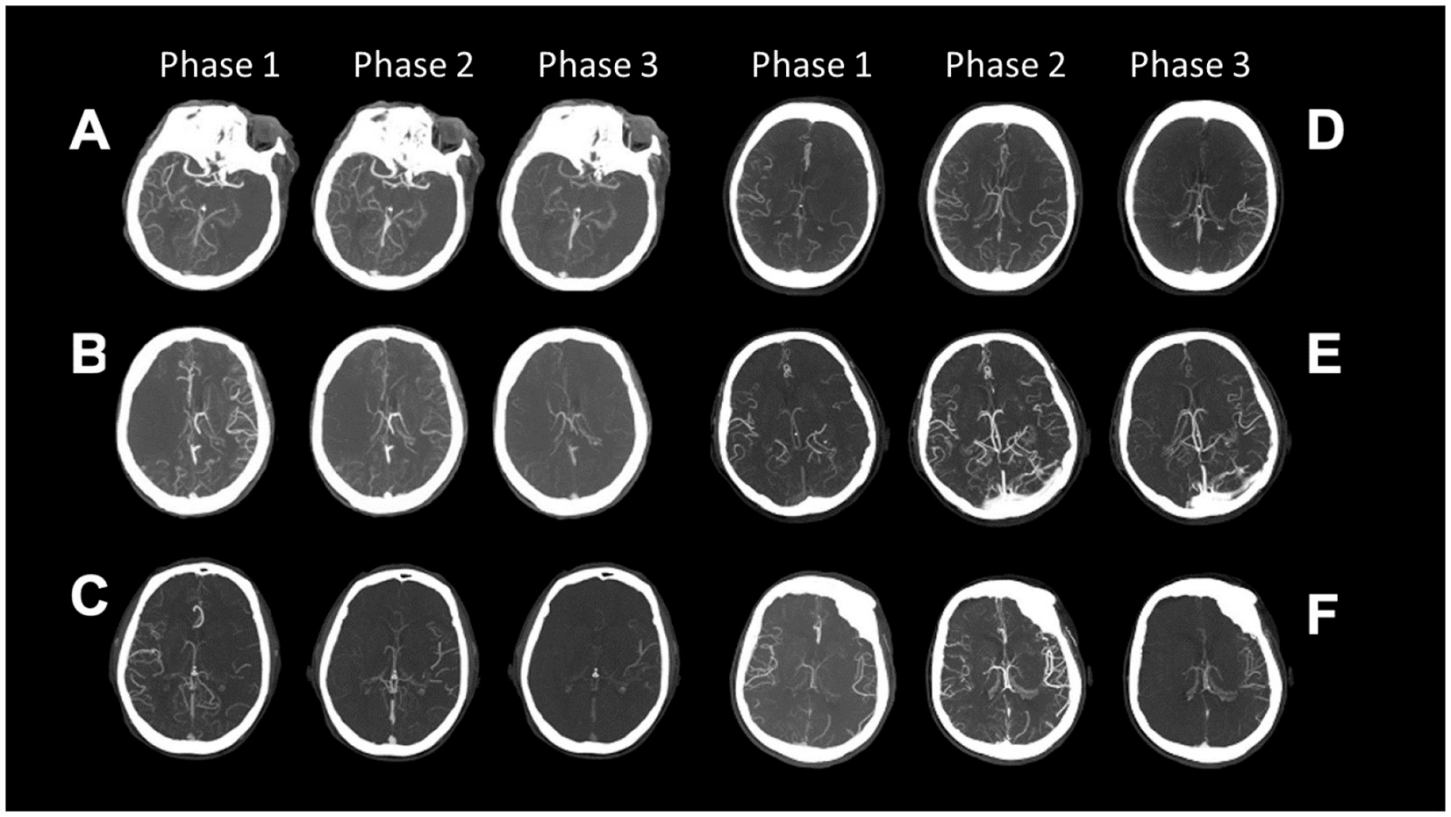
Collaterals on three phases of multiphase CT Angiography classified according to the Menon Grading Score (A–F) is Grade 0–5; (Table 1).
